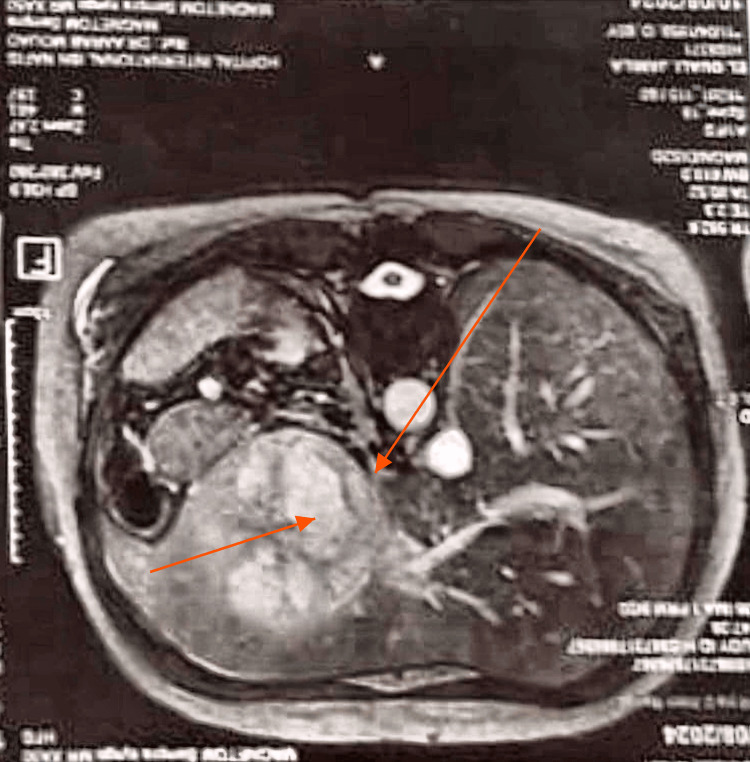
Representative axial T2-weighted MRI image demonstrating a multiloculated hepatic abscess with heterogeneous content (arrow), representing the systemic infectious focus responsible for hematogenous endogenous dissemination. Microbiological analysis of the drainage fluid isolated *Klebsiella pneumoniae*. MRI: magnetic resonance imaging
